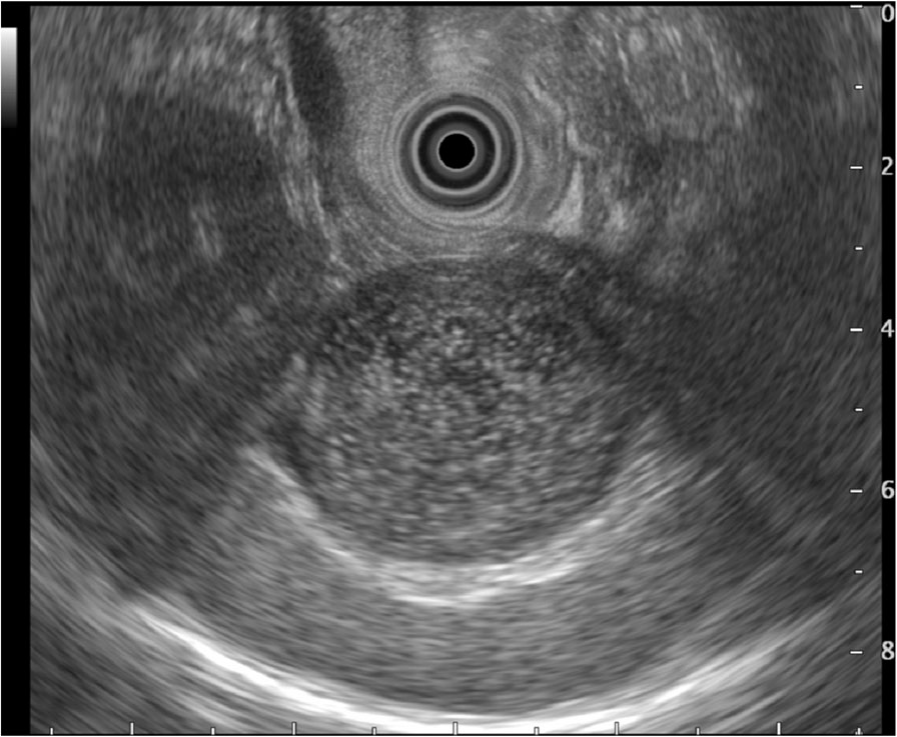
Endoscopic ultrasound showing the tumor, which appears smooth with a marginal edge, characterized by echo with high homogeneity, and the presence of viscous mucus was suspected
